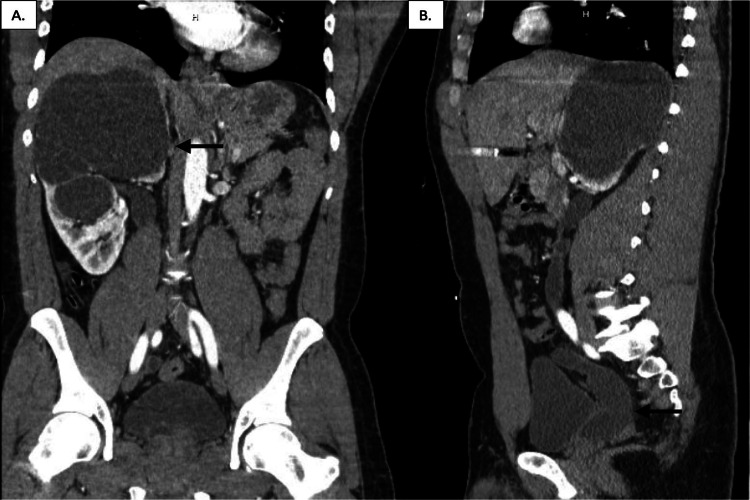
CT abdomen and pelvis without contrast prior to surgery (A) Coronal view of the right kidney revealing severe hydronephrosis of the right kidney with cystic changes. Notice the dilation of the ureter from the upper pole indicated by the black arrow. (B) Sagittal view of severely hydronephrotic right ureter inserting into the posterior prostatic urethra (black arrow).
